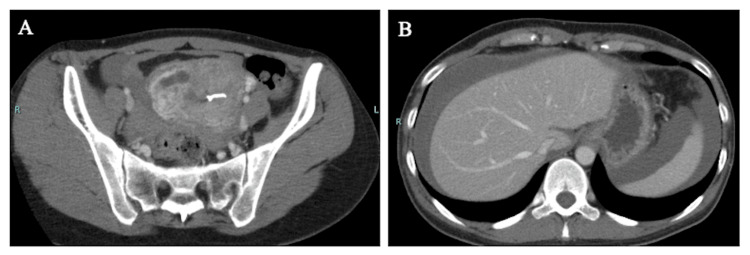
Contrast-enhanced CT of the upper abdominal and pelvic region (A) A 5 cm mass was noted on the right side of the uterus, and LNG-IUS was noted in the uterine lumen; (B) Ascites had accumulated up to the upper abdomen.
